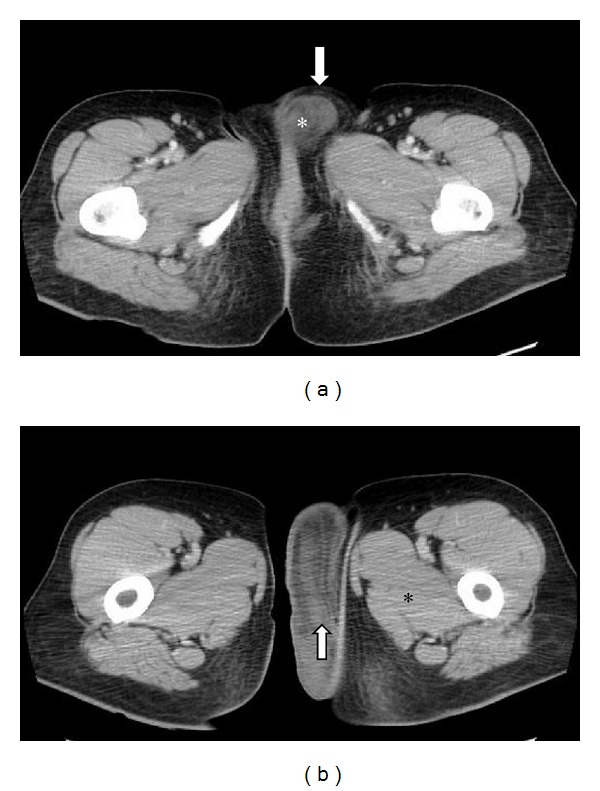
(a) Axial noncontrast CT image showing vulvar skin edema (arrow) and the suspected aggressive angiomyxoma at its anterior origin. (b) Axial CT image with contrast showing the posterior extent of the aggressive angiomyxoma with the swirled appearance (arrow) and the attenuation difference between it and surrounding skeletal muscles; less attenuation compared to skeletal muscle (asterisk).
